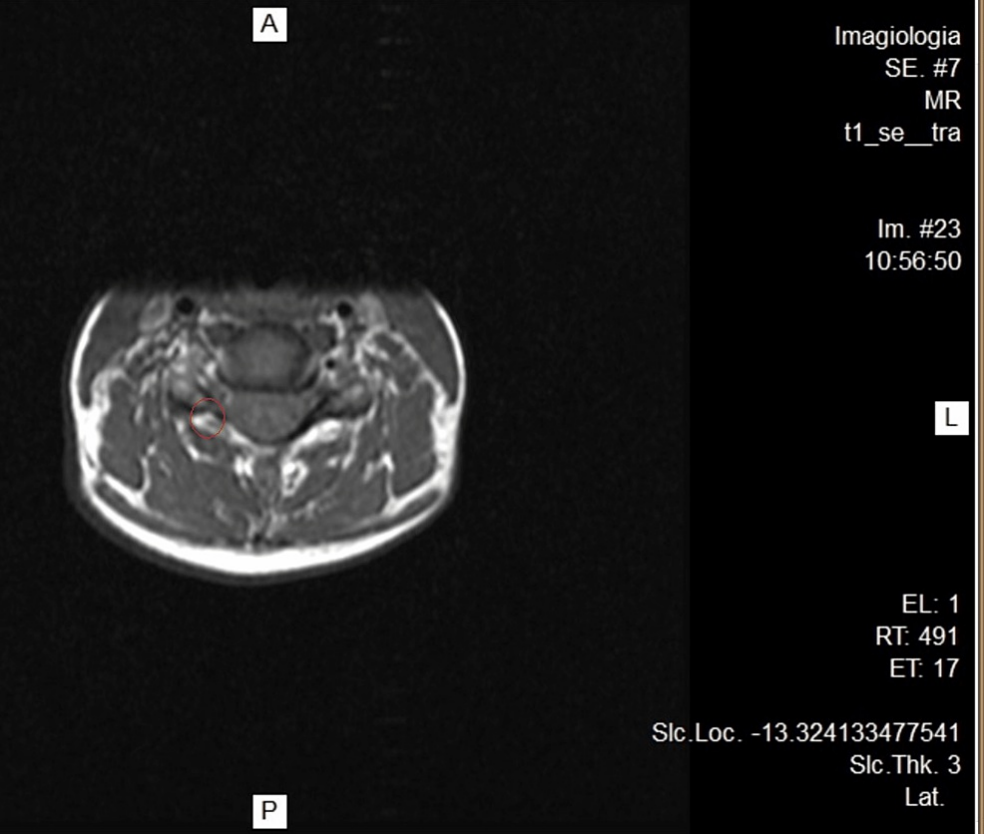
Cervical MRI. C5-C6, with less expression on the left
